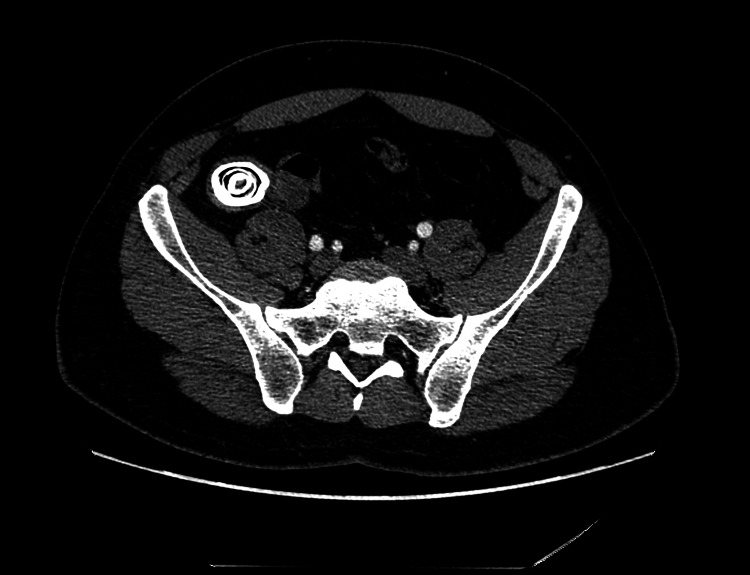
Axial postcontrast CT shows the lamellated appearance of the giant appendicolith within the right lower quadrant, with mild appendiceal mural thickening, indicative of mild acute appendicitis. CT: computed tomography
